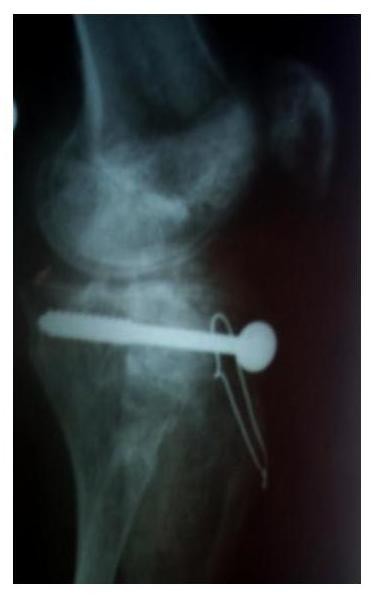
Lateral x-ray of the same patient after hybrid external fixator removal.
